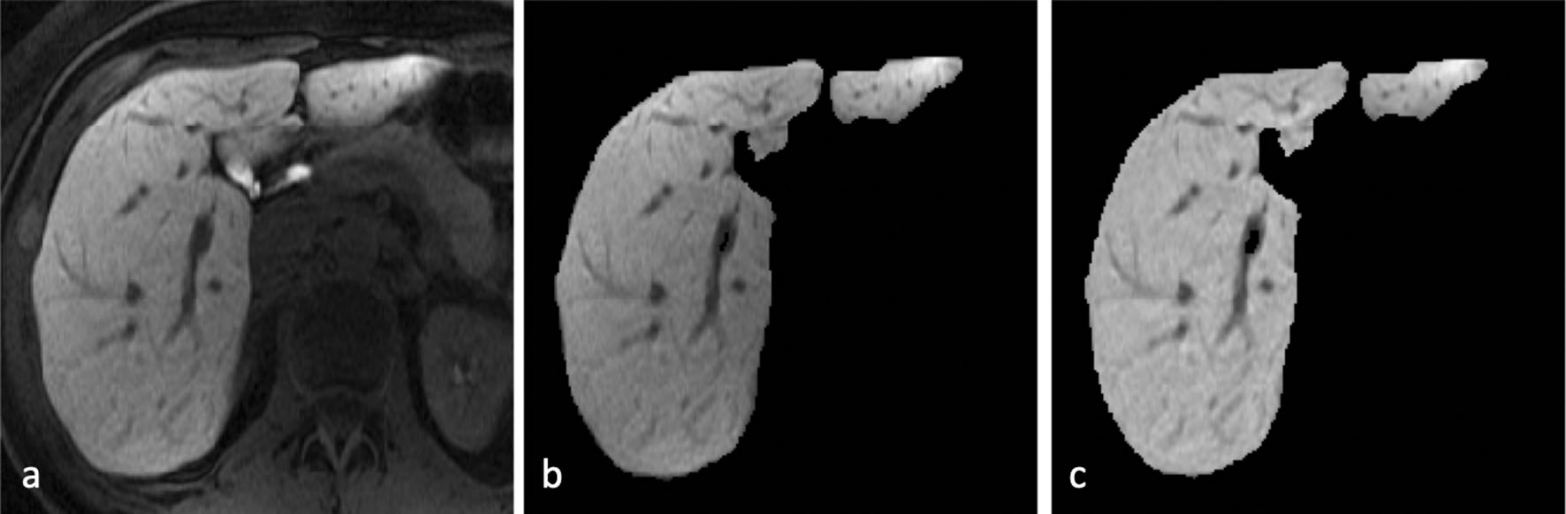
An example of liver MRI preprocessing (a) raw image (b) liver segmentation (c) noise reduction and background inhomogeneity correction.
